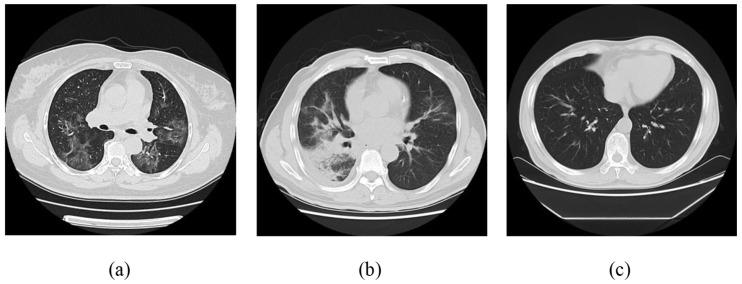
Different sections of the transverse on CT image; (a) on the novel coronavirus; (b) on influenza A viral pneumonia (IAVP); (c) on a healthy candidate who is not infected with the virus.
